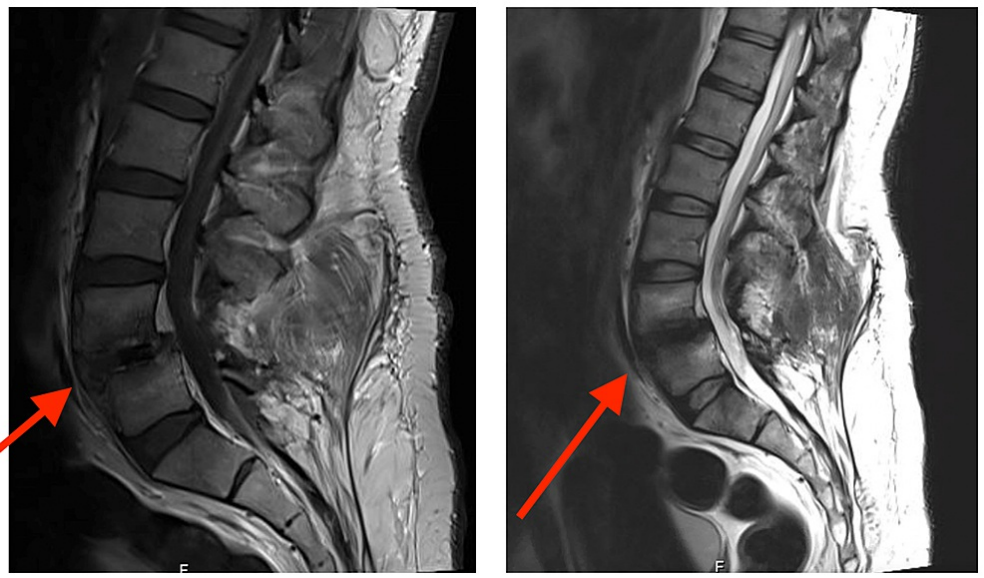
pre-revision MRI of lumbar spine showing post-operative changes and mild anterior translation of L4 over L5 with associated bilateral facet arthropathy (red arrows), with combined lytic and degenerative 1st-degree anterolisthesis.
